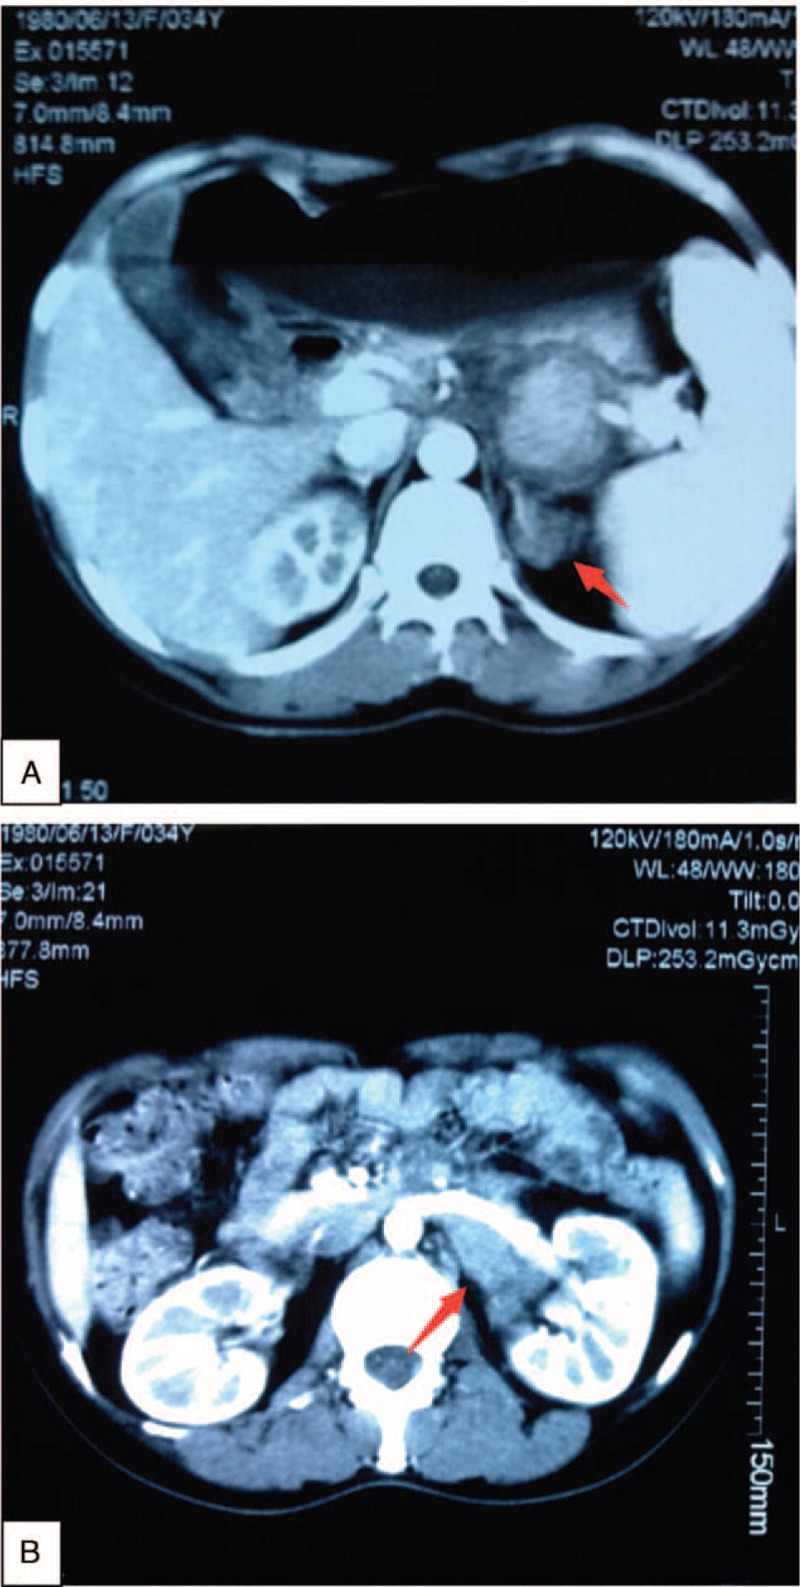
Contrast-enhanced computed tomography of abdomen reveals 2 conspicuous unclear mass located in the left adrenal area and left renalis of approximately 4.5 cm × 3 cm and 3 cm × 2 cm, respectively (A-B).
